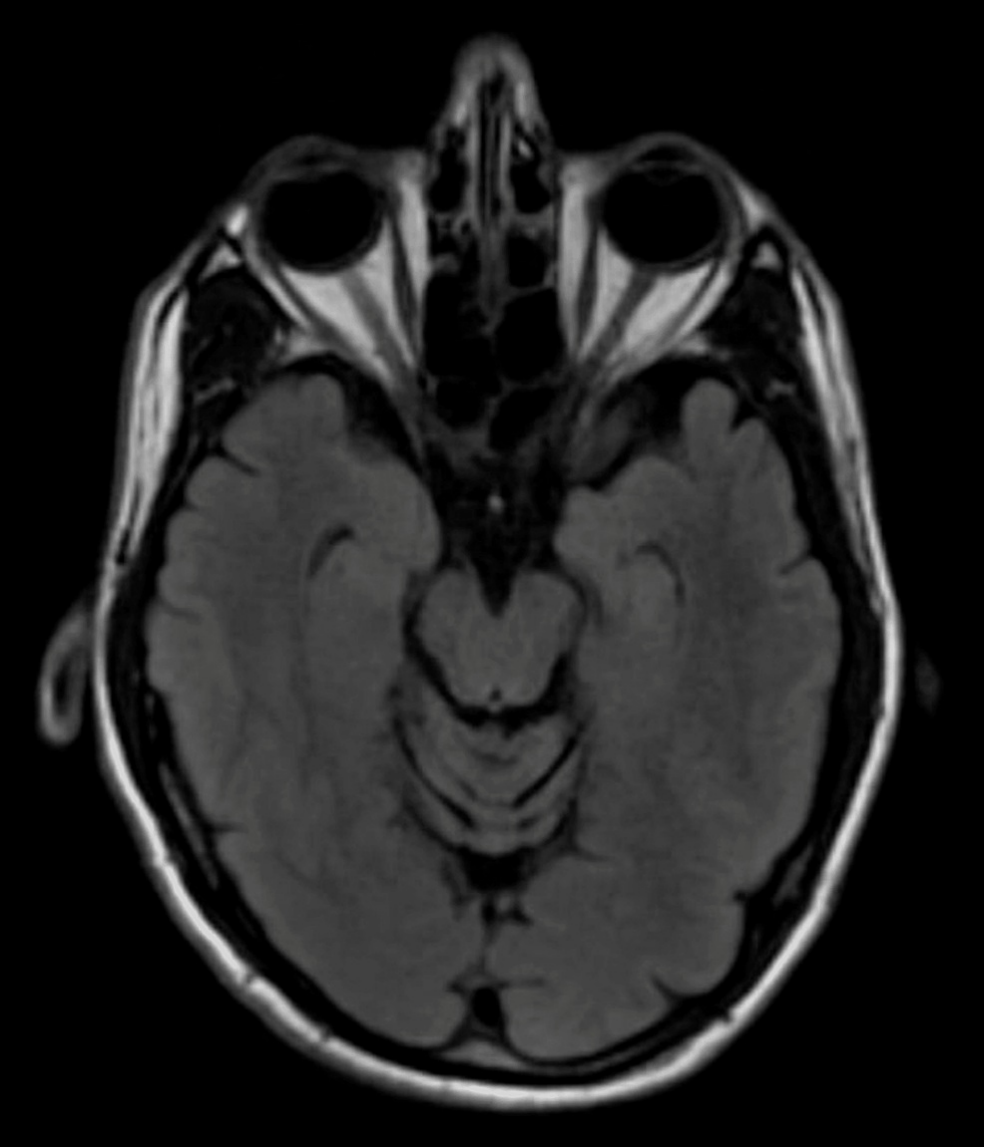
MRI of the brain showing no muscular or optic nerve edema
